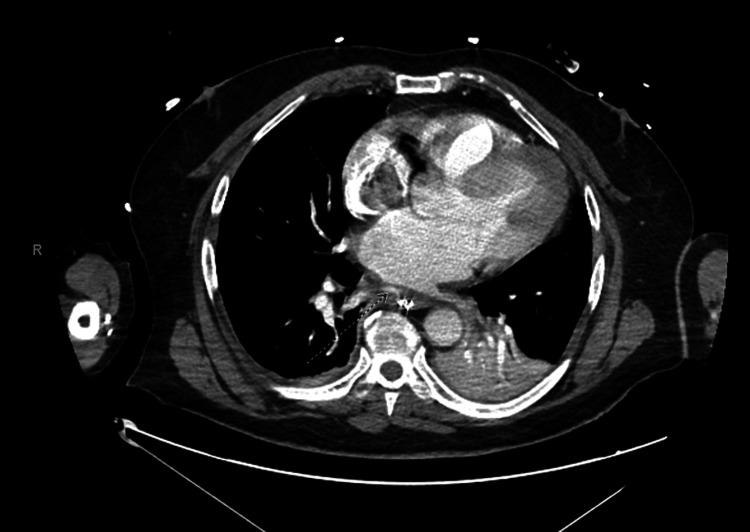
Computed tomography angiography of chest/abdomen/pelvis with contrast showing the atrial esophageal fistula
